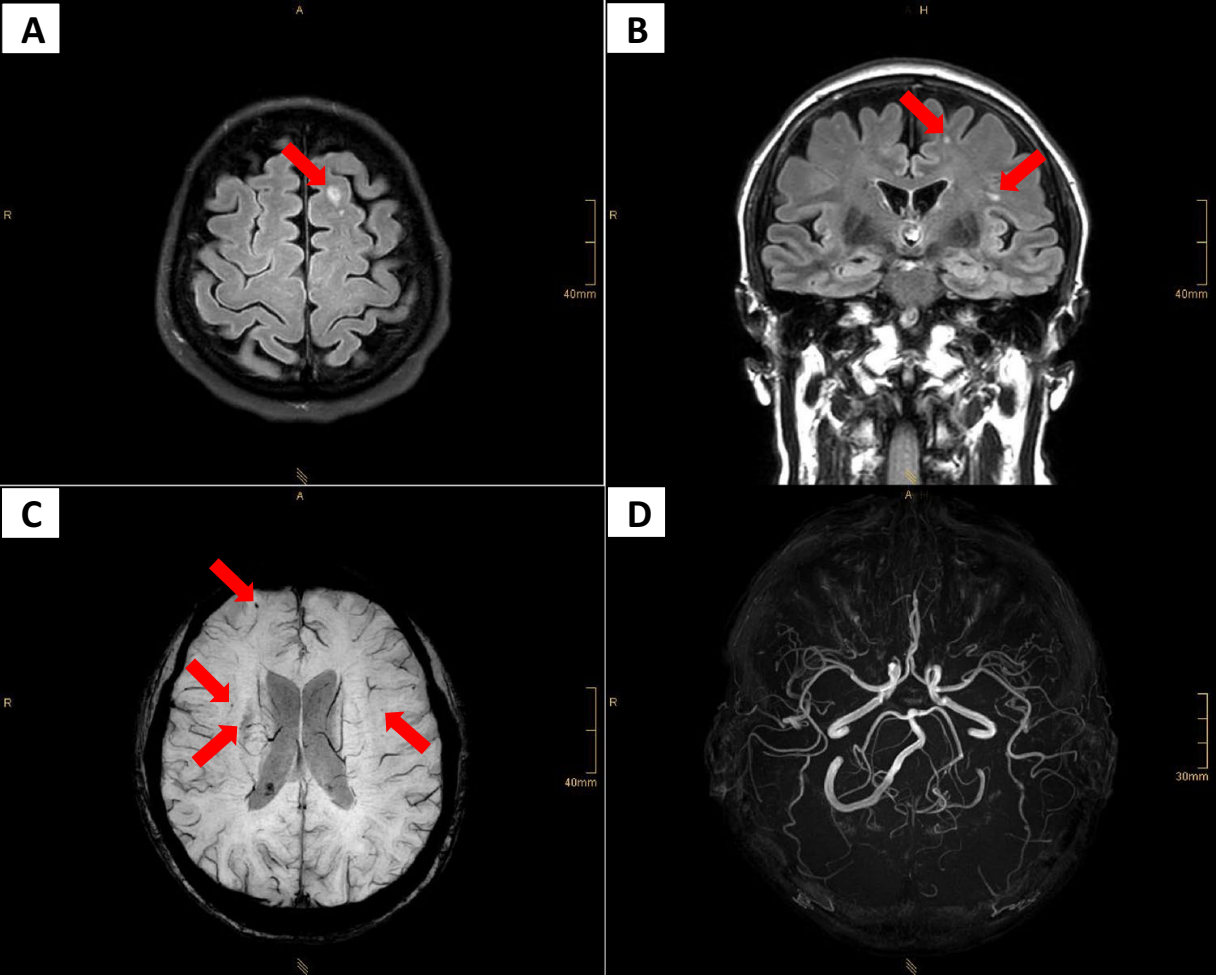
Brain-MRI performed in August 2022; Methodology: The examination was performed on the 3Tesla-MRI with weight-adjusted intravenous contrast medium administration (CMA, gadolinium). Sequences obtained: FLAIR*,* axial scan; DWI*,* ADC map, axial scan*;* SWI axial scan; axial FLAIR 3D scan; Time-of-Flight MR angiography; axial T2tse scan; 3D T1*-*weighted sequences after CMA with coronary and axial reconstructions. Results and conclusions: Although the cerebral vasculature is well-structured and intact without any detectable pathological changes (D), small vessels clearly show signs of micro-angiopathy; several lacunar microinfarction zones, white matter hyper-intensities and micro-haemorrhages (A, B, C) are evident; no any recent ischemic lesions have been recorded
